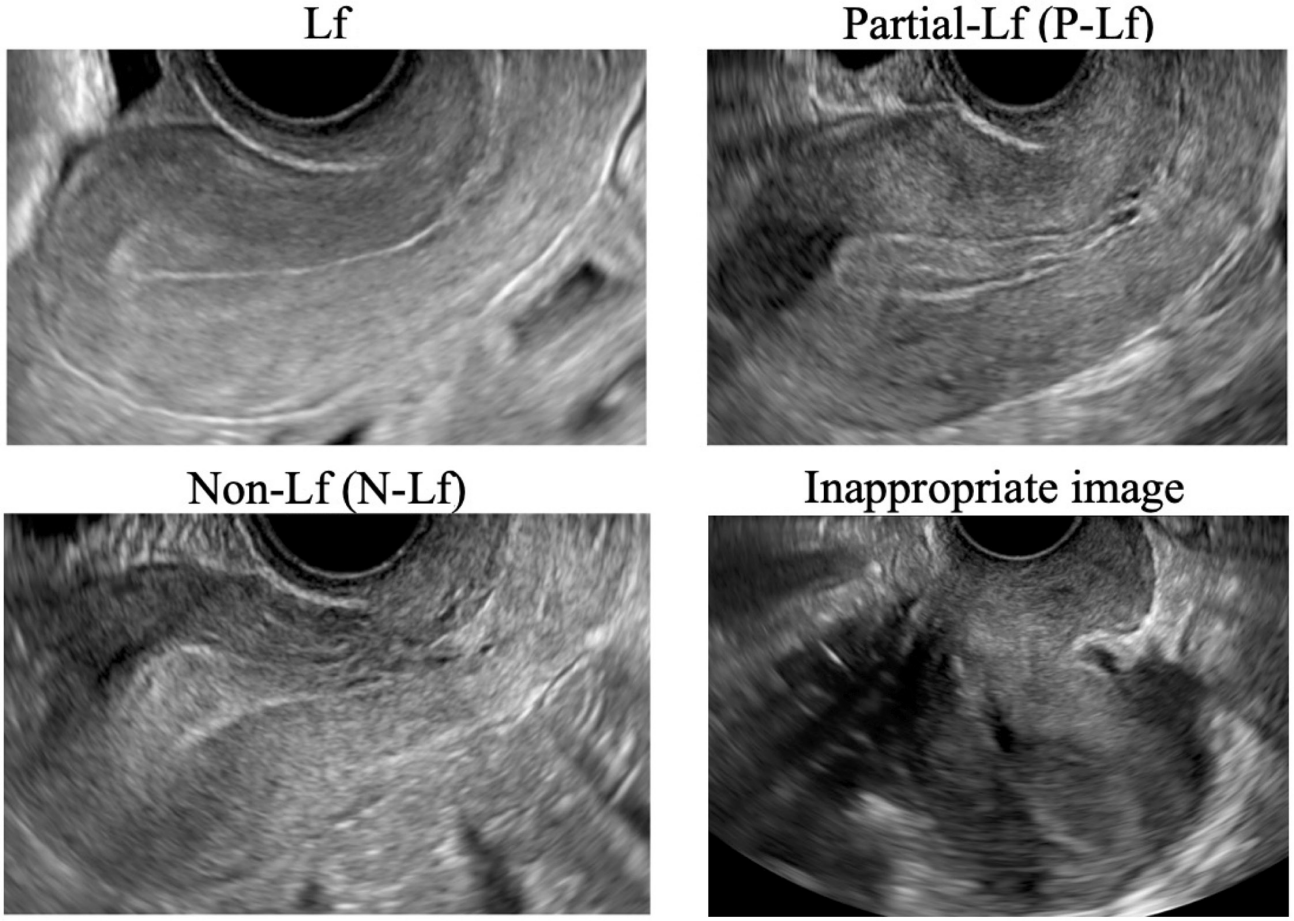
Endometrial pattern classification: Representative ultrasound images. Leaf pattern (Lf): Central echogenic line present and continuous (unbroken) along its full length. Partial‐Lf: Central echogenic line present but discontinuous (broken) in places. Non‐Lf: Central echogenic line of leaf pattern cannot be clearly distinguished. Inappropriate: Endometrial pattern was indistinguishable because the image was captured with the major axis of the uterus parallel to the transvaginal probe.
